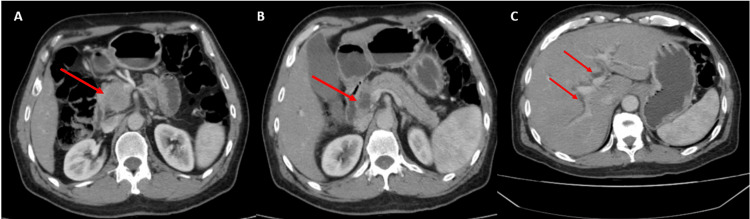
CT abdominal sections of the patient. Pancreatic head processus measuring 51×48×35 mm in large axes (arrows) which can be primitive or secondary (A) responsible for biductal biliary expansion of Wirsung at 4 mm and the main biliary tract at 12.5 mm (B) with dilation of the intra-hepatic biliary tract (C).
